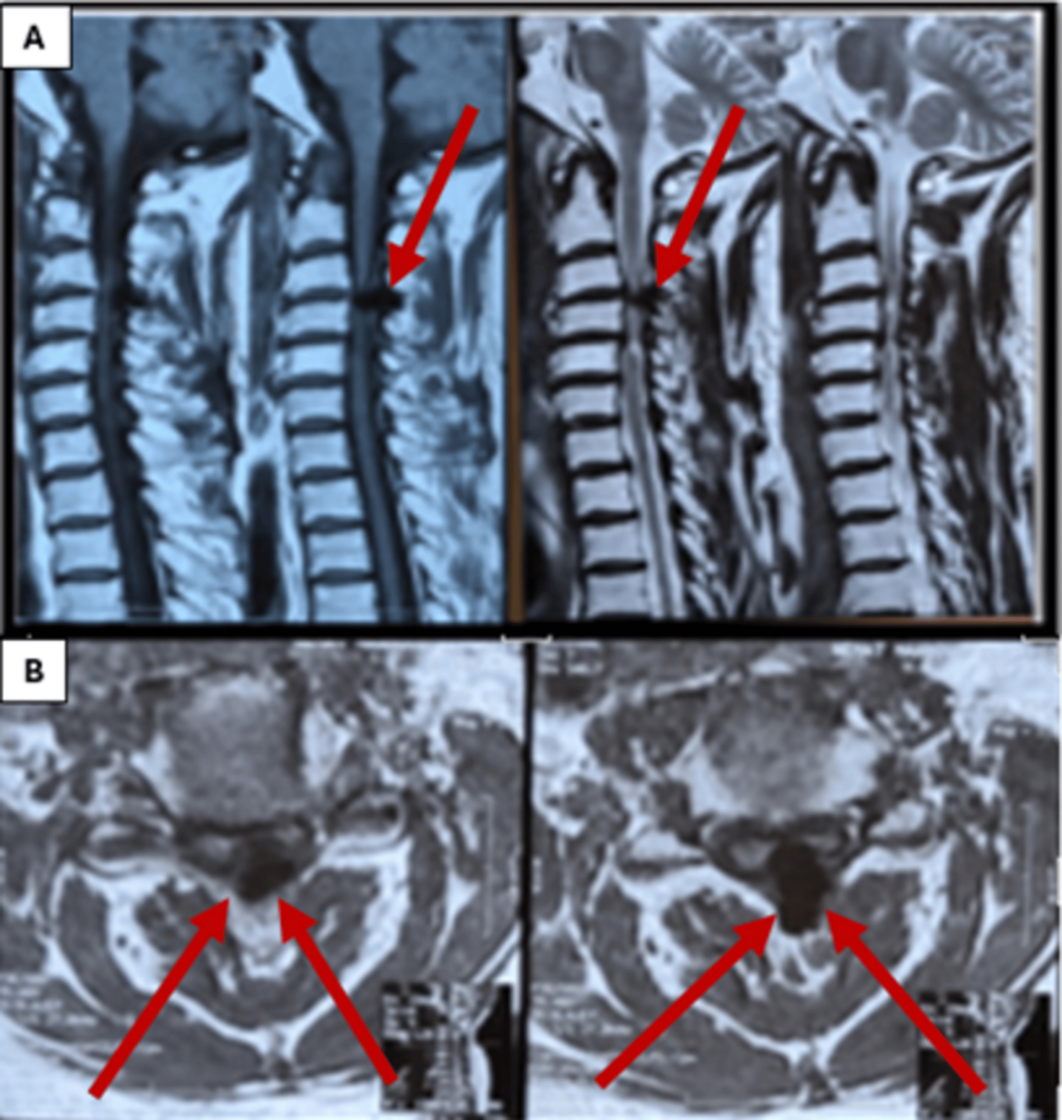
Preoperative cervical spine MRI (A) Sagittal view and (B) axial view showing posterior cord compression by a nodular lesion in the ligamentum flavum at the C3-C4 and C4-C5 levels. The low signal intensity on both T1- and T2-weighted images indicates a calcified lesion. Additionally, intramedullary high signal intensity (myelomalacia) is present at the affected levels.
